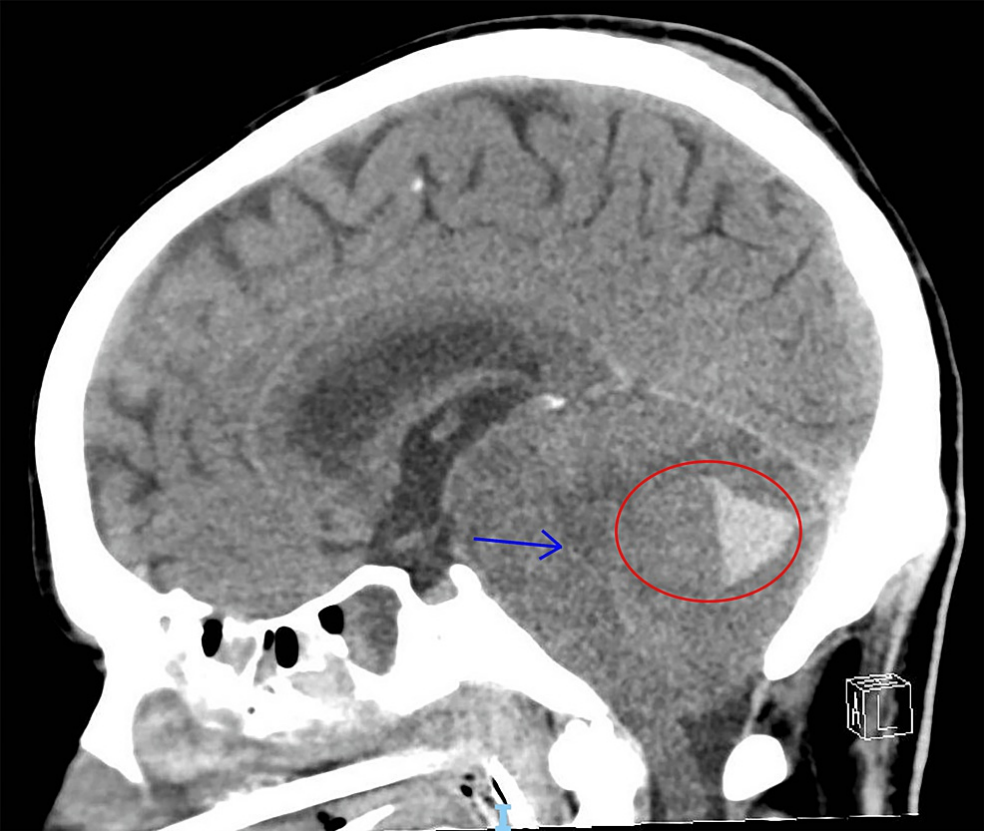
Final CT Head Final CT imaging after the patient was stabilized enough for transportation. Shown is a mid-sagittal slice. Imaging demonstrates enlarging left cerebellar parenchymal hemorrhage (red circle, measured at 5.7 cm x 1.8 cm) with effacement of the basal cisterns and fourth ventricle and brainstem compression (blue arrow).
